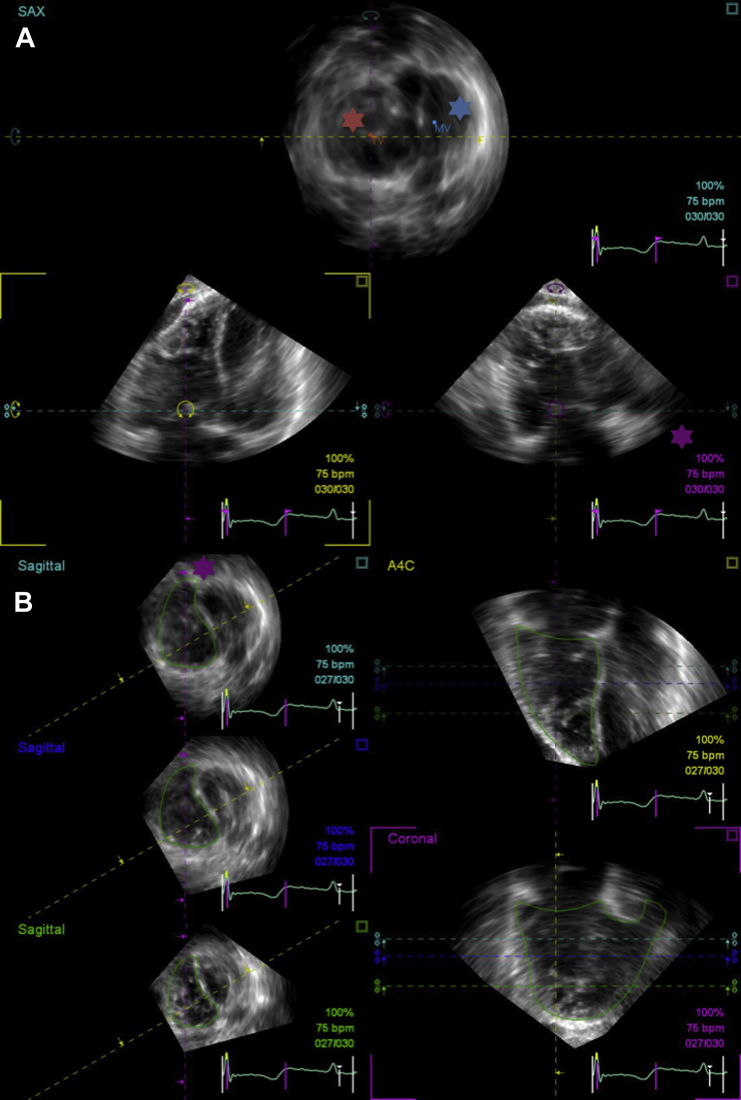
Example of the stepwise process of RV reconstruction by 3DE. (A) The cavity area is optimized in the three orthogonal views, and landmarks are identified (mitral valve indicated by the *blue asterisk*, tricuspid valve indicated by the *red asterisk*). Note the RVOT seen in the coronal view (indicated by the *purple asterisk*). (B) Having traced endocardial borders in three orthogonal views, the semiautomated border tracking results are displayed for inspection. Note how the purple guideline (indicated by the *purple asterisk*) bisects the tricuspid valve and RVOT in the short-axis views. This corresponds to the coronal RV reconstruction (*highlighted*), with clearly delineated RV inflow and outflow portions. *A4C*, Apical four-chamber.
